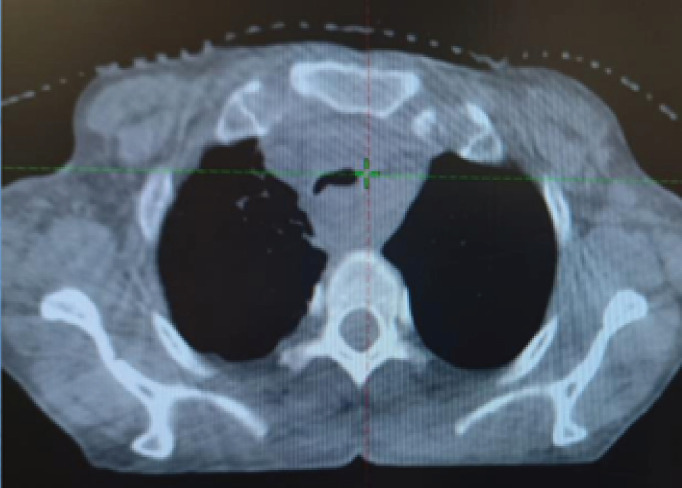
CBCT at first consolidative RT fraction showing regression in the tracheal compression.
